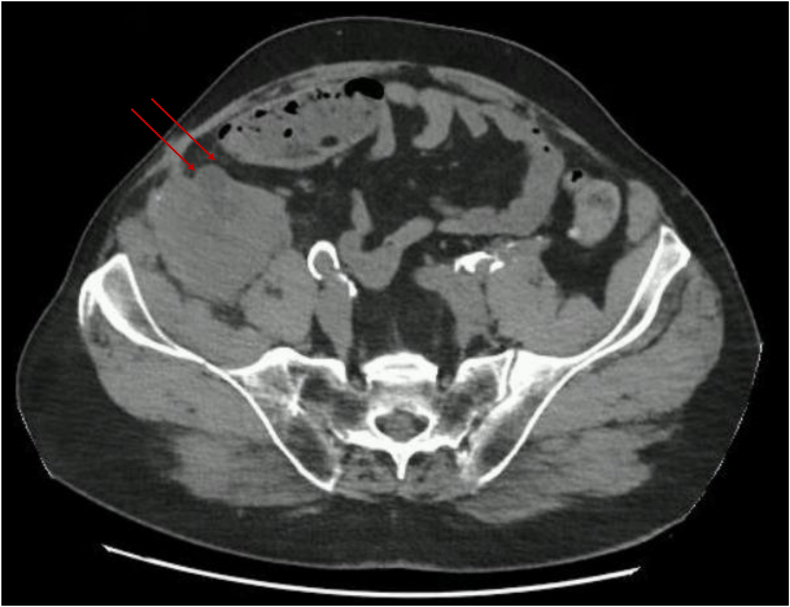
CT-scan from the last follow-up (2 months after the double-J stent removal) Renal graft pelvis (arrows), without pyelocaliceal dilatation or adjacent collection.
